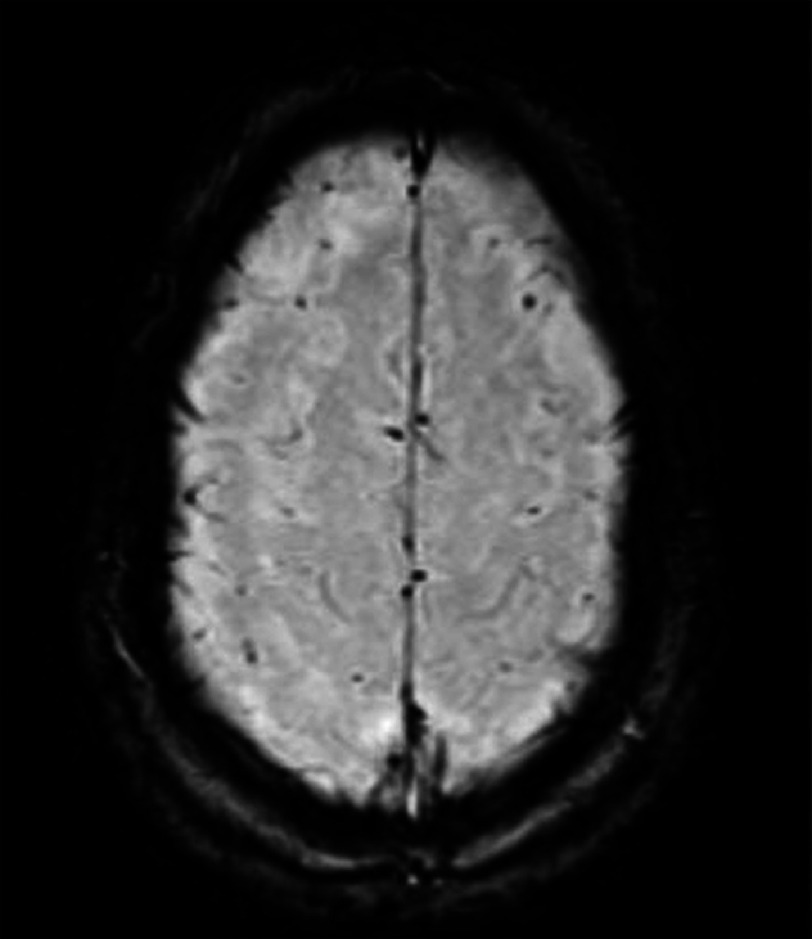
MRI of the brain demonstrating diffuse hemosiderin deposits reflecting remote hemorrhagic episodes, most likely secondary to arteritis. There is no evidence of intracranial hemorrhage, extra-axial collection, mass effect, or midline shift.
